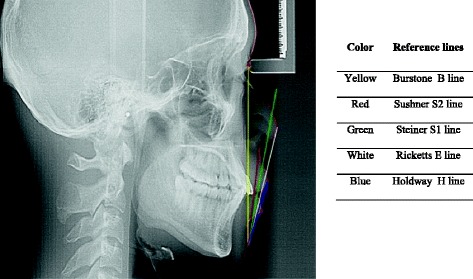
Lateral cephalometric radiograph representing the five reference lines.
